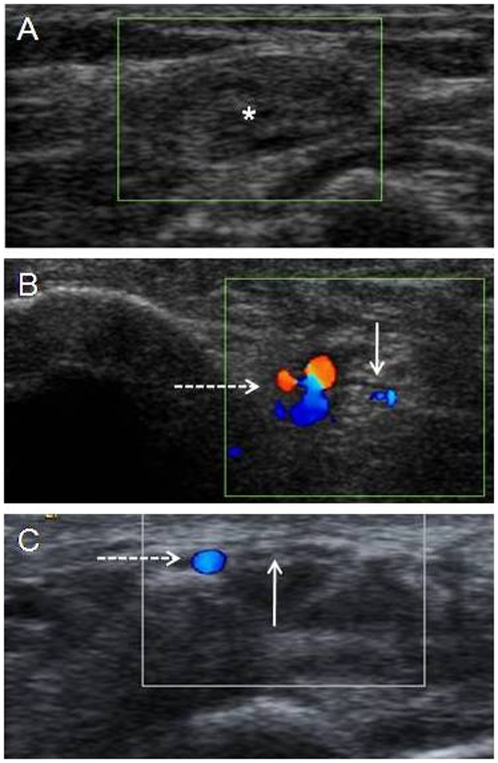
Ultrasonography and color Doppler images of peripheral nerves of leprosy patients. A transverse scan of an enlarged ulnar nerve indicated by an asterisk (CSA = 45 mm^2^) from BT leprosy patient with type 1 reaction showing no endoneural blood flow signals; B colour Doppler image of a right ulnar nerve in a BT patient (CSA = 51 mm^2^) undergoing type 1 leprosy reaction, showing increased endoneural and perineural blood flow signals (demarcated by solid arrow in a rectangular box, dashed arrow in a box shows a blood vessels) suggestive of acute neuritis; C colour Doppler image of a median nerve (shown by solid arrow) in a BT patient (CSA = 9 mm^2^) undergoing type 1 leprosy reaction with persistent artery (shown by dashed arrow), showing no endoneural flow signal.
